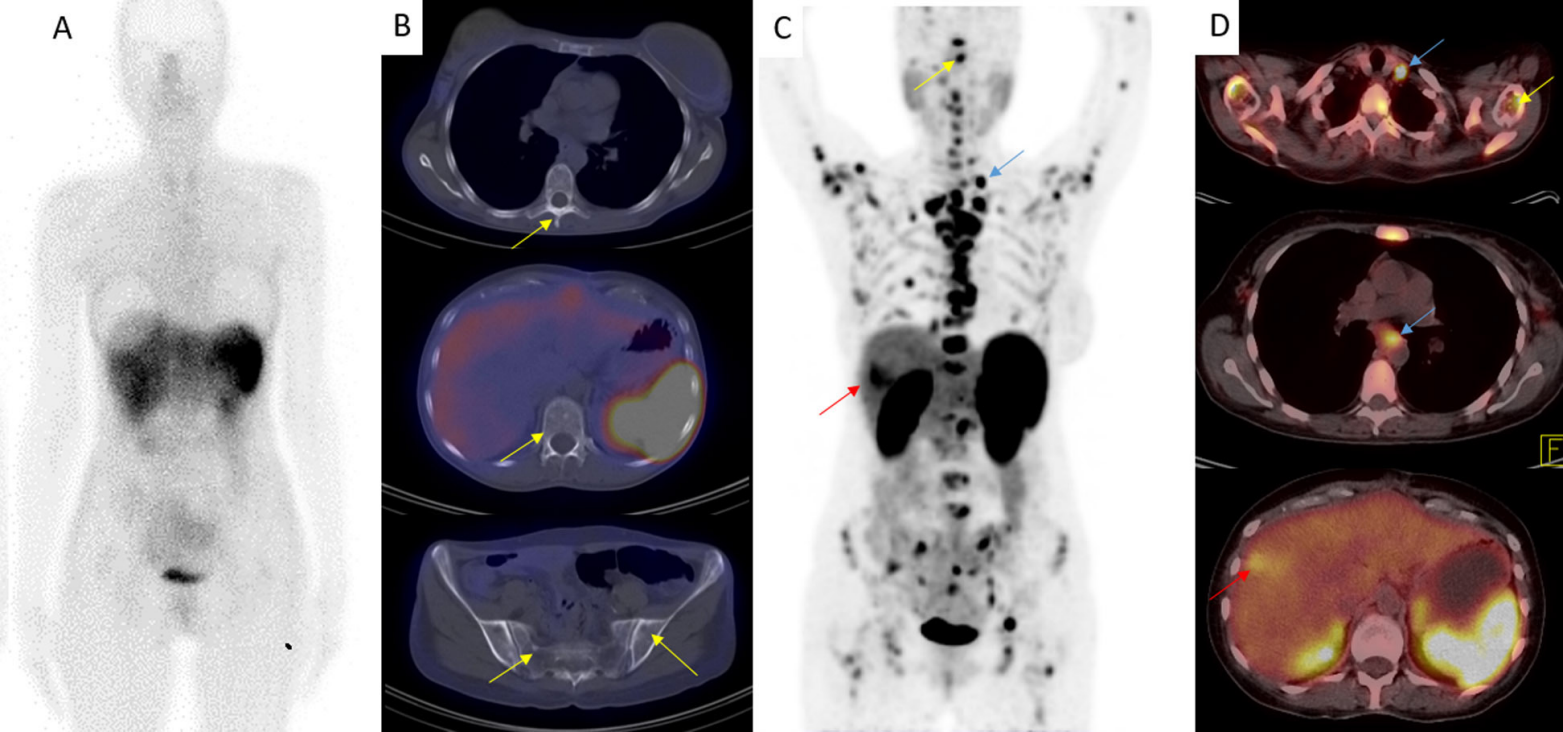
A 40-year-old female with history of metastatic carcinoid tumor, undergone partial pancreatectomy and liver transplantation two years ago. She was evaluating for increasing levels of chromogranin A. (A) Planar and (B) transaxial ^99m^Tc-Octreotide SPECT/CT showed physiologic radiotracer distribution without any abnormal focus of uptake. Lytic/sclerotic lesions were apparent on low-dose CT (yellow arrows). (C) Whole body and (D) transaxial ^68^Ga-DOTATATE PET/CT revealed widespread bone metastases (yellow arrows), as well as metastatic lymph nodes (blue arrows) in the left supraclavicular and mediastinal regions. Also, evidence of fatty liver with a focal spared parenchyma (red arrow) is visualized
